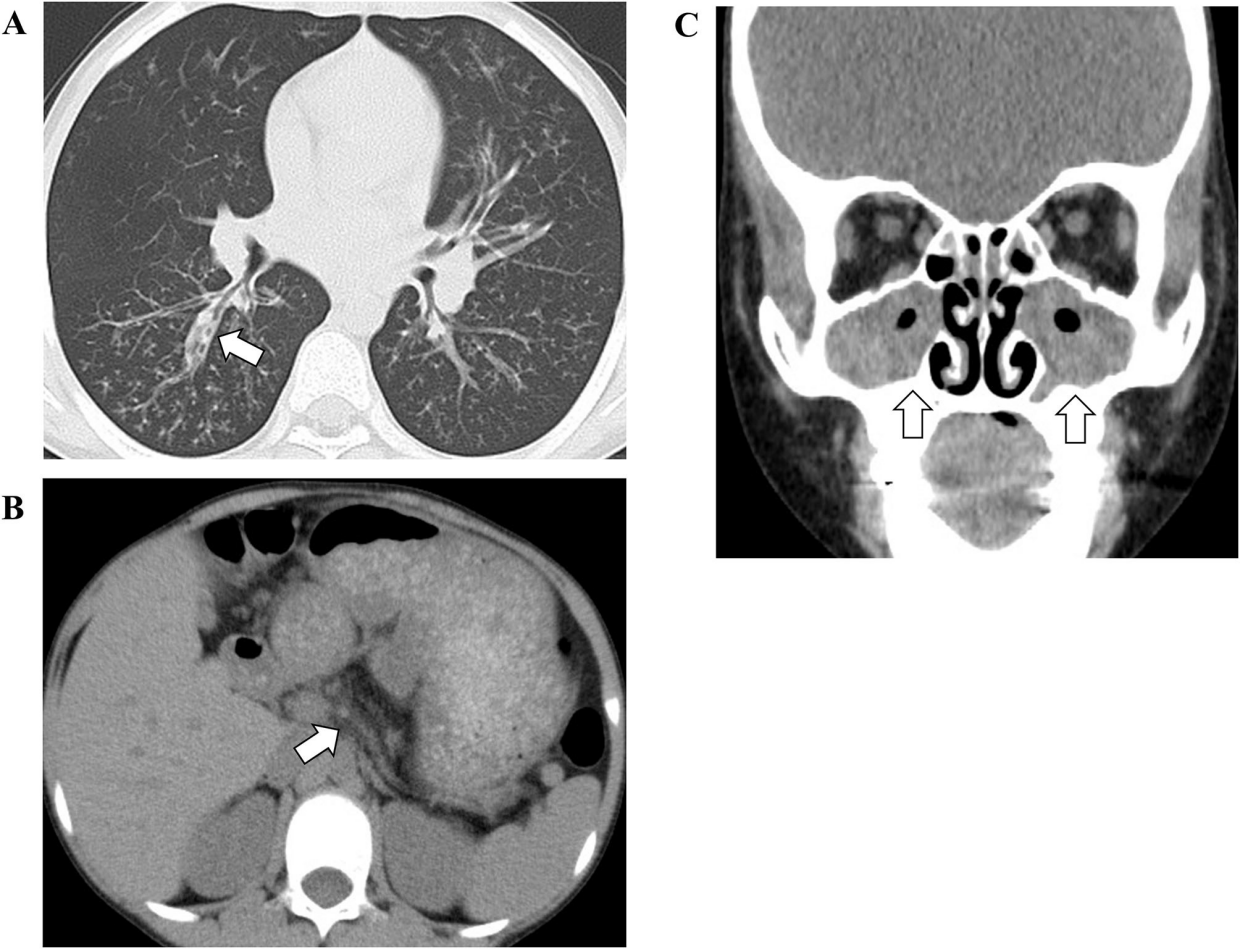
The findings of chest (A), abdominal (B), and facial (C) computed tomography. The white arrow in (A) indicates thickened bronchial walls and bronchiectasis. The white arrow in (B) indicates an atrophic pancreas. The white arrows in (C) indicate gas-fluid level in the paranasal sinuses and gas bubbles within the fluid.
